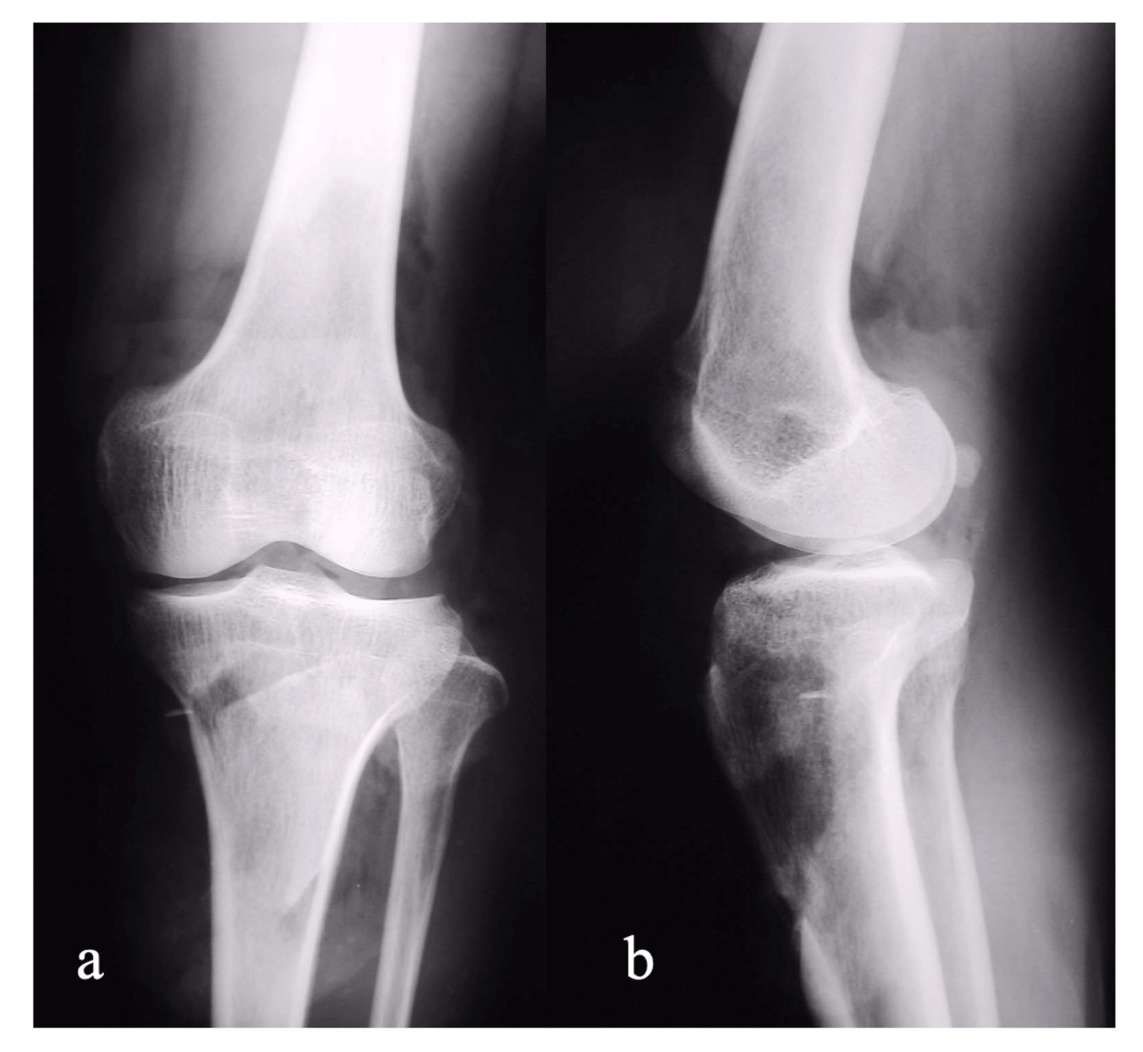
Plain X-ray of the right knee. A: AP view
